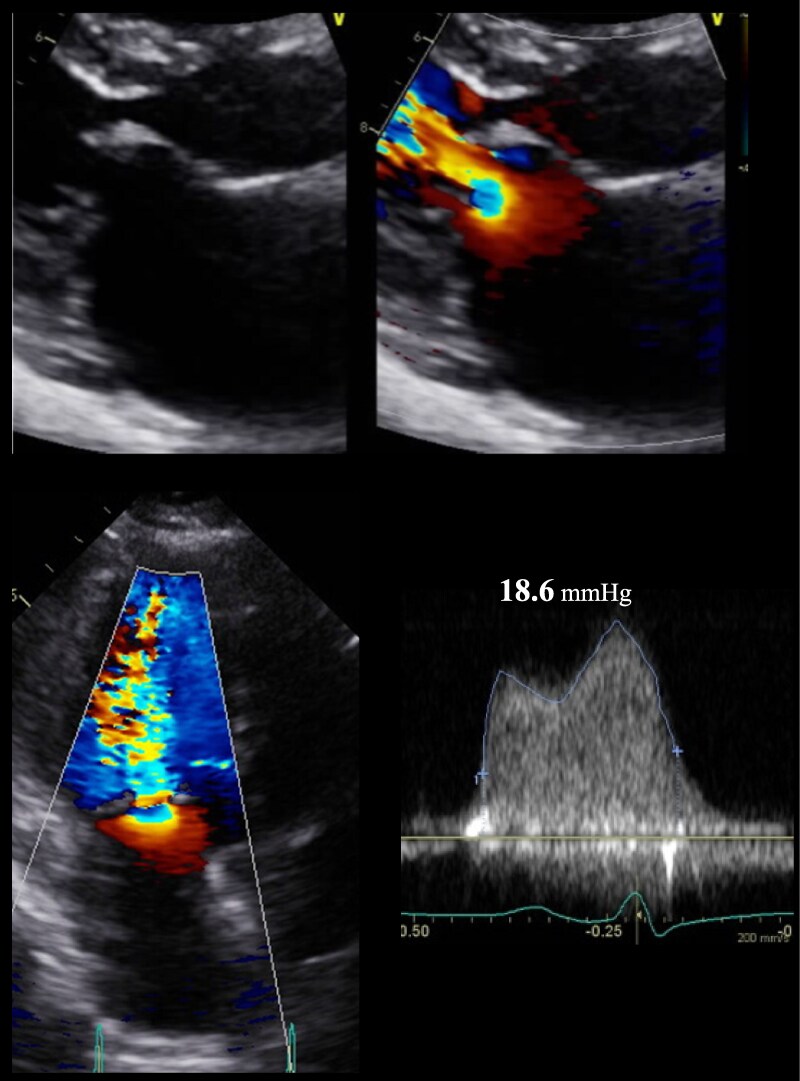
Transthoracic echocardiography at admission for congestive heart failure revealed severe mitral valve stenosis, with a mean transvalvular pressure gradient of 18.6 mmHg across the mitral valve.
